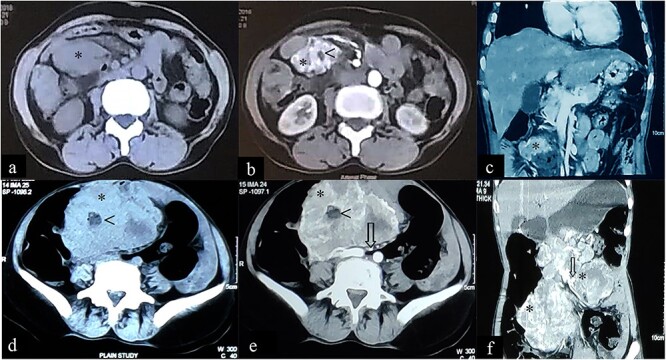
Case 1: CT imaging of extragastrointestinal stromal tumor. (a) Non-contrast axial, (b, c) contrast enhanced axial and coronal reformatted CT images of abdomen demonstrate heterogeneously enhancing confluent mass (*) in peritoneal cavity anterior to the iliac vessels with central area of necrosis (<) peripherally displacing mesenteric vessels (arrow) and bowel. (d–f) Non-contrast axial, contrast enhanced axial and coronal reformatted CT images of abdomen of same patient after 1-year follow-up showing increased interval size of the lesion (*).
